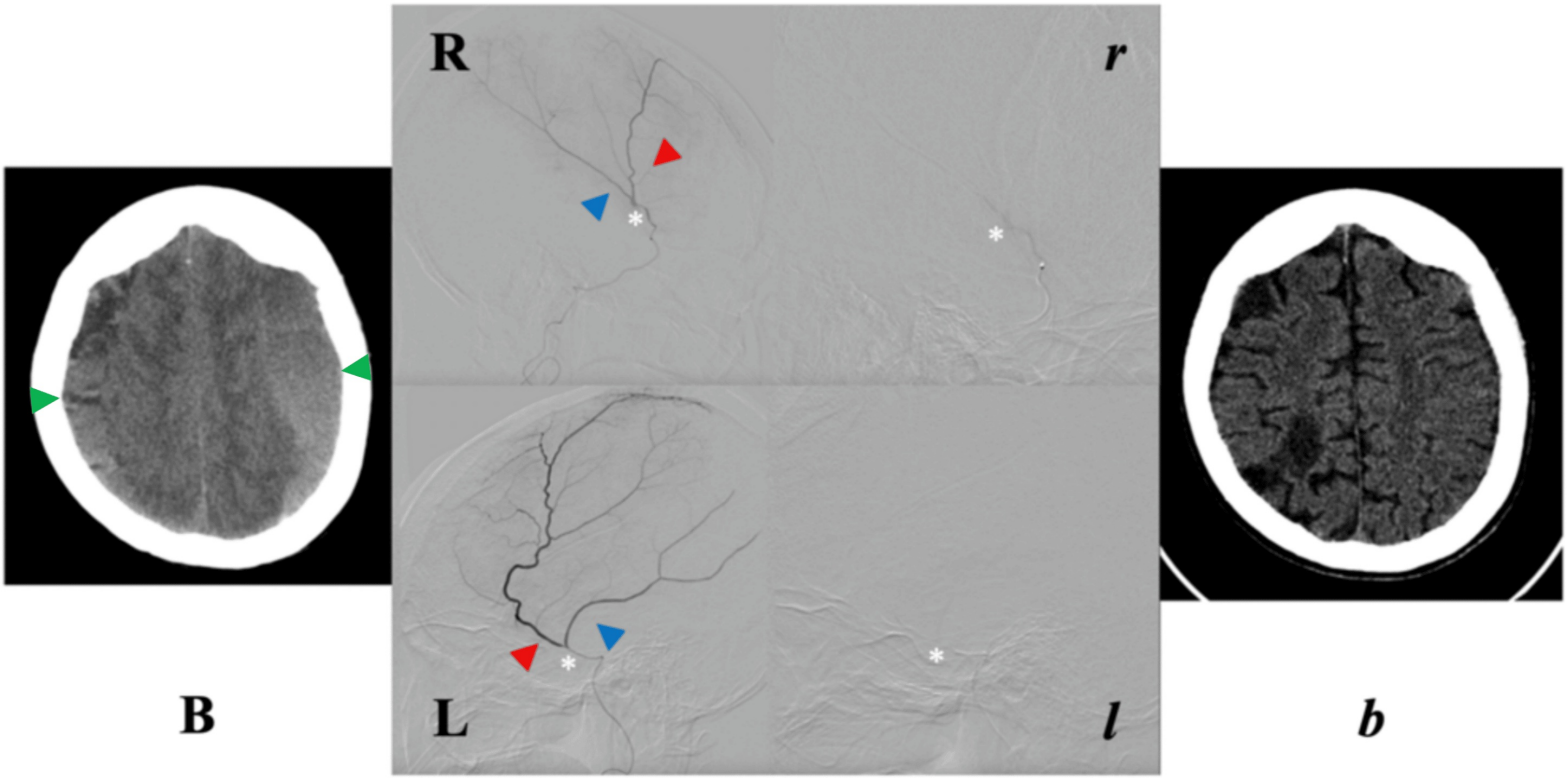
Case B. Pre-EMMA (B) and respective post-EMMA (b) cranial NCCT axial views at levels of maximum CSDH thickness. Pre-EMMA angiographies in lateral projections (R, right; L, left) show the course of anterior and posterior MMA branches, and post-EMMA obliteration of these vessels (r, right; l, left). Angiographic projections correspond to contiguous NCCTs. EMMA, embolization of the middle meningeal artery; L, left; MMA, middle meningeal artery; NCCT, non-contrast computed tomography; R, right. Green arrowhead, chronic subdural hematoma; red arrowhead, anterior branch; blue arrowhead, posterior branch; white asterisk, MMA bifurcation point.
